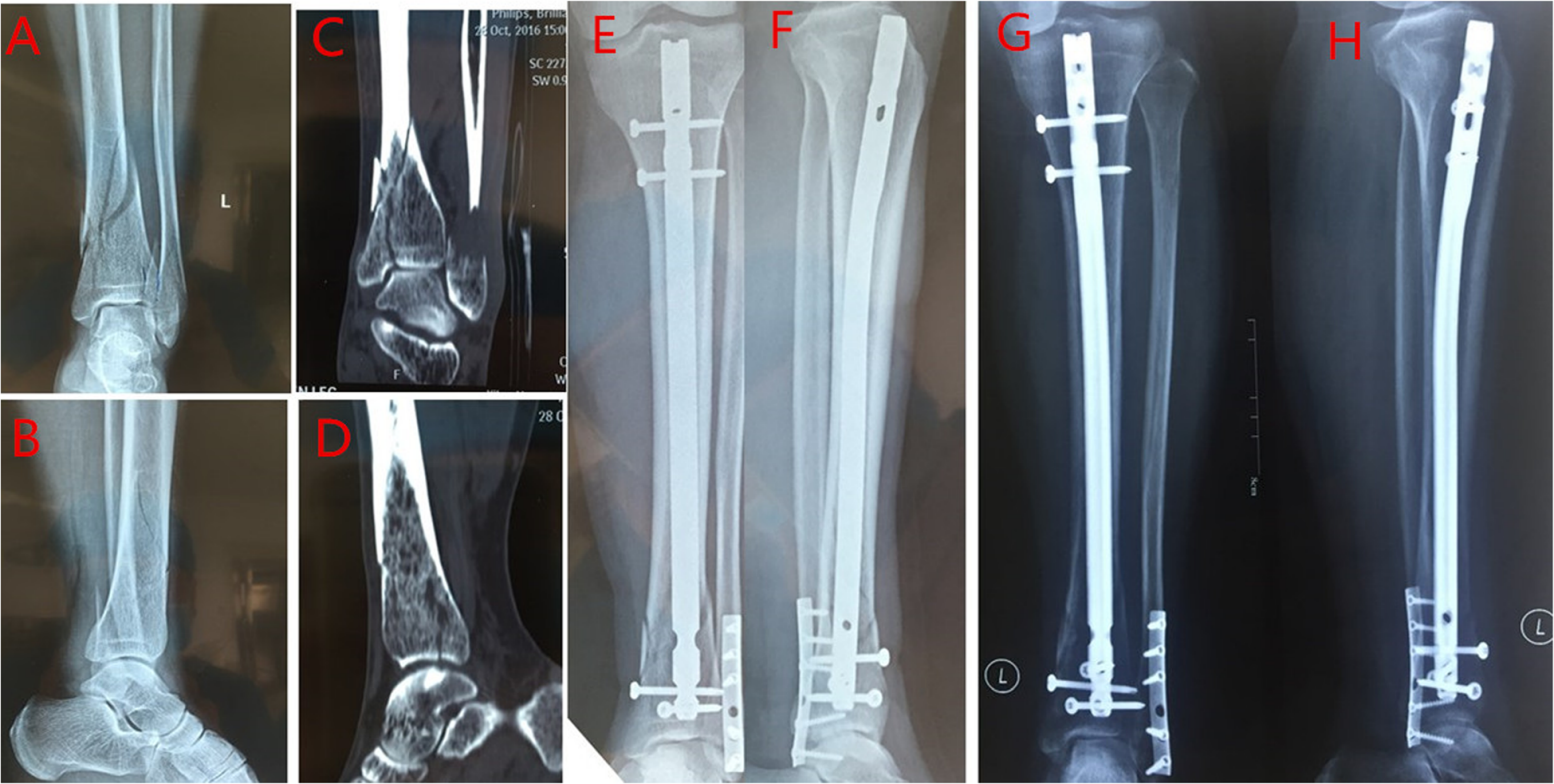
Radiographs of a case of union after closed reduction using the suprapatellar approach were presented. a, b Preoperative anteroposterior (AP) and lateral views. c, d Computed tomography views, fracture involving the ankle joint. e, f AP and lateral views postoperatively. g, h AP and lateral views 6 months postoperatively
